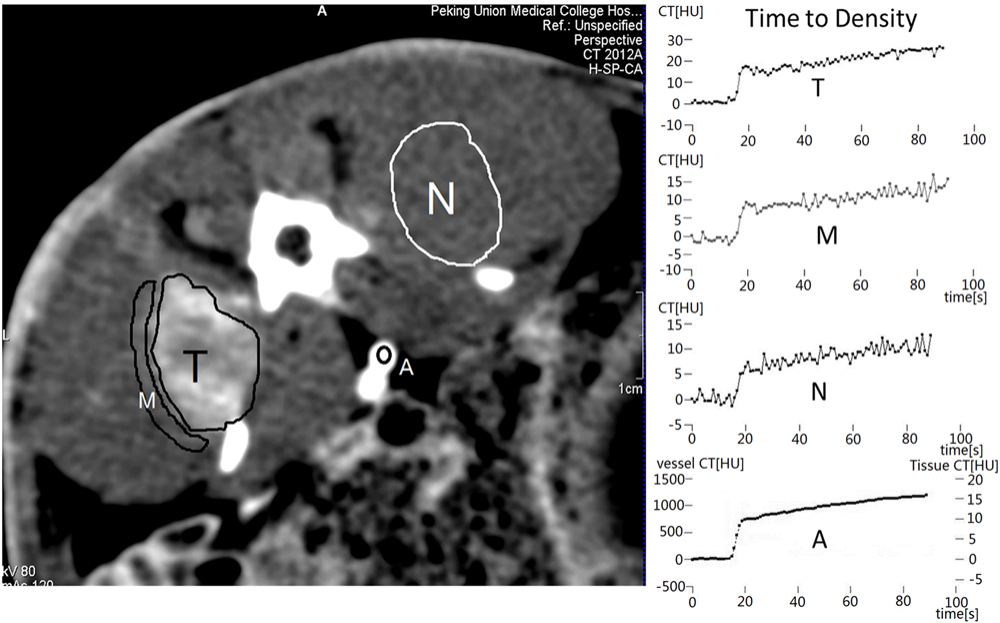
Axial perfusion CT image and time density curve corresponding to the regions of interest: center of the aorta as an arterial input reference (A), around entire tumor (T), around an area of muscle tissue immediately adjacent to the tumor (M), and random location in the normal muscle tissue distant from the tumor (N).
